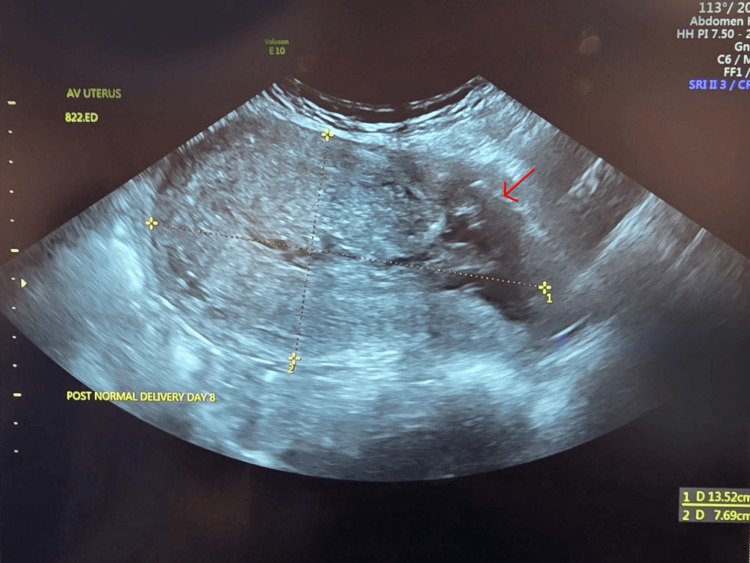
Pelvic ultrasound showing a large caesarean section scar defect (measures: 28 mm), with a large hematoma extending from the lower endometrial cavity through the open caesarean scar externally in between the uterus and urinary bladder.
